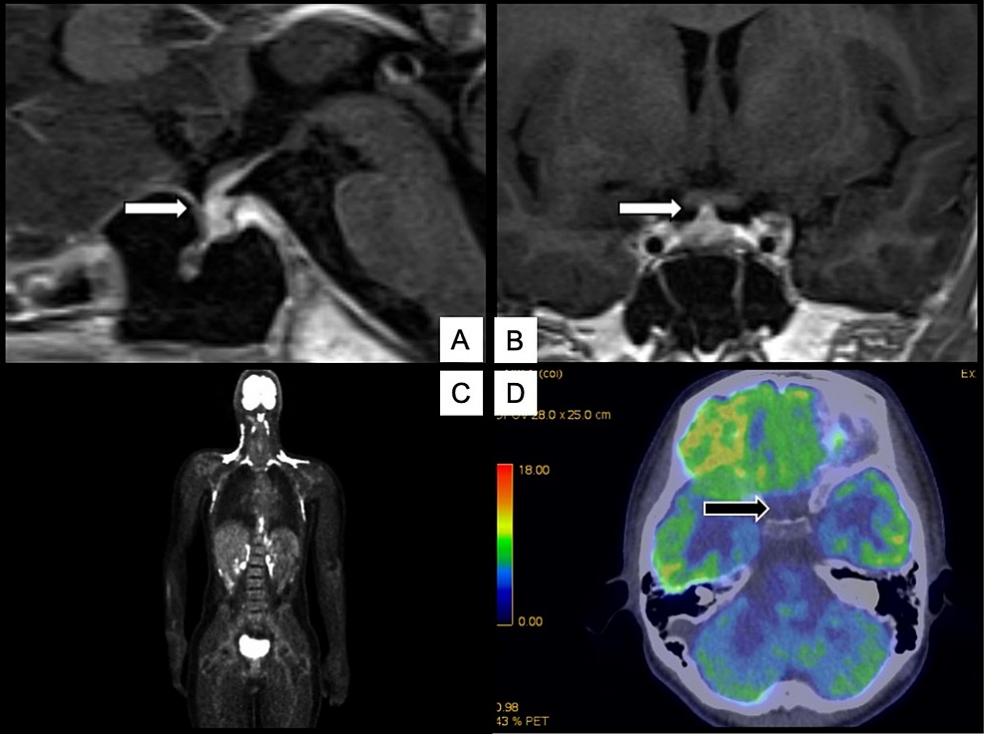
Enhanced T1-weighted MRI and FDG-PET The white arrow indicates the pituitary and stalk; no enlargement is observed (A, B). In the following two images, FDG-PET of the entire body (C) and brain (D) is shown. No accumulation of fluorodeoxyglucose (FDG) is observed. The black arrow indicates that there is no accumulation in the pituitary and stalk.
